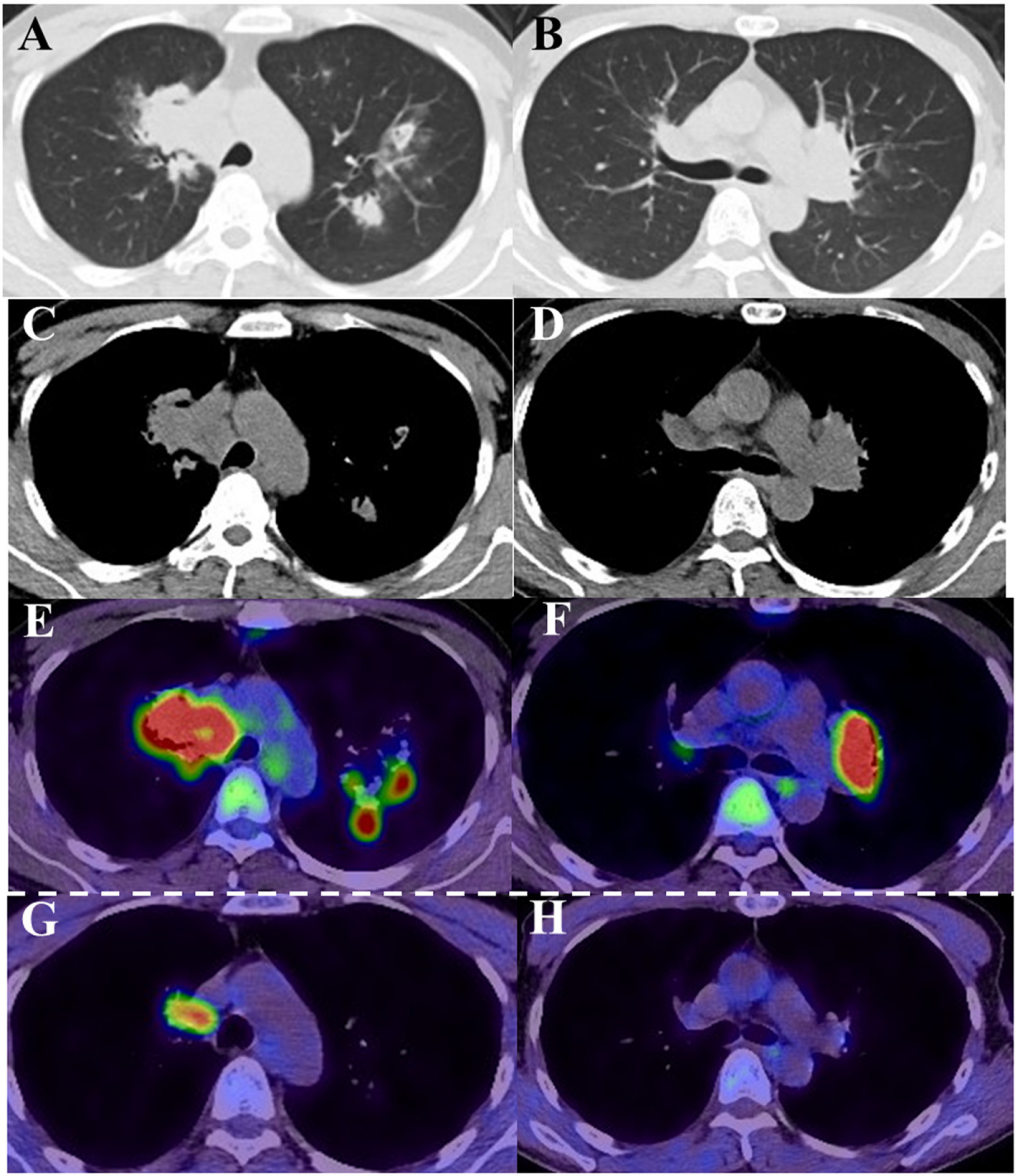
Chest computed tomography and ^18^ F-fluorodeoxyglucose positron emission tomography-computed tomography findings. a–d A computed tomography scan performed five months after starting cART showed bilateral infiltrates in the upper lobes of the lungs and mediastinal and bilateral hilar lymphadenopathy. e, f The ^18^ F-fluorodeoxyglucosepositron emission tomography-computed tomography (^18^ F-FDG PET/CT) scan performed five months after starting cART showed intense accumulation of fluorodeoxyglucose (FDG) around the infiltrates and the mediastinal and bilateral hilar lymphadenopathy (maximum standardized uptake value: 18.42). g, h The ^18^ F-fluorodeoxyglucose positron emission tomography-computed tomography (^18^ F-FDG PET/CT) scan performed one year after starting anti-mycobacterial chemotherapy showed a decreased uptake of FDG when compared to the scan performed five months after starting combined antiretroviral therapy (cART). Moreover, a reduction in FDG uptake was observed in the area of the lesions with the exception of a right-lower paratracheal lymph node (station #4R; maximum standardized uptake value: 6.24)
